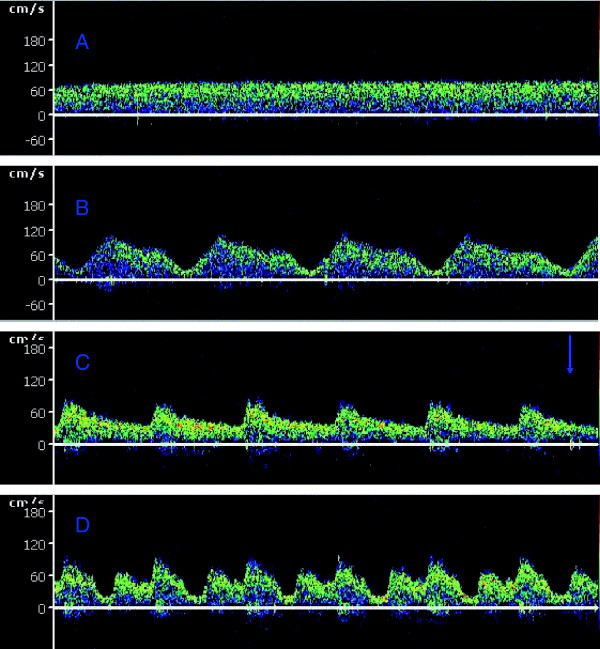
Typical middle cerebral artery flow waveform obtained by transcranial Doppler ultrasonography during extracorporeal membrane oxygenation. A, B, N group. C, D, P group. (A) The CBFV while IABP is turned off in severe cardiac failure. (B) The CBFV while ECMO was combined with IABP support in severe cardiac functional failure. (C) The CBEV while IABP is turned off in spontaneous cardiac function. (D) The CBFV while ECMO was combined with IABP support in spontaneous cardiac function.
